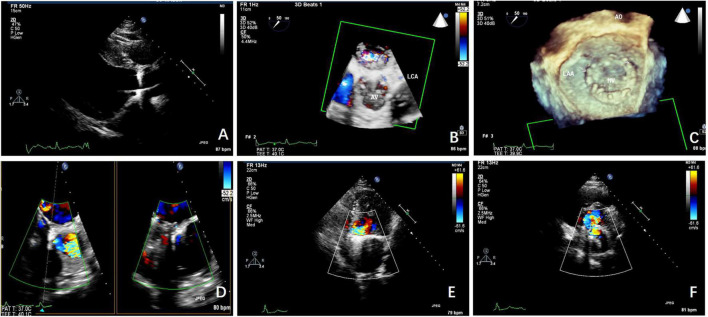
The TTE and transesophageal echocardiographic (TEE) scans taken before the procedure. (A) TEE shows the aortic paravalvular leakage (PVL) before the procedure. (B) 3-Dimensional (3D)-TEE shows the aortic PVL before the procedure. (C) 3D-TEE shows the mitral PVL before the procedure. (D) The mitral PVL as seen on the TEE scan. (E) The mitral PVL as seen on the TTE scan. (F) The mitral PVL was as seen on TTE.
